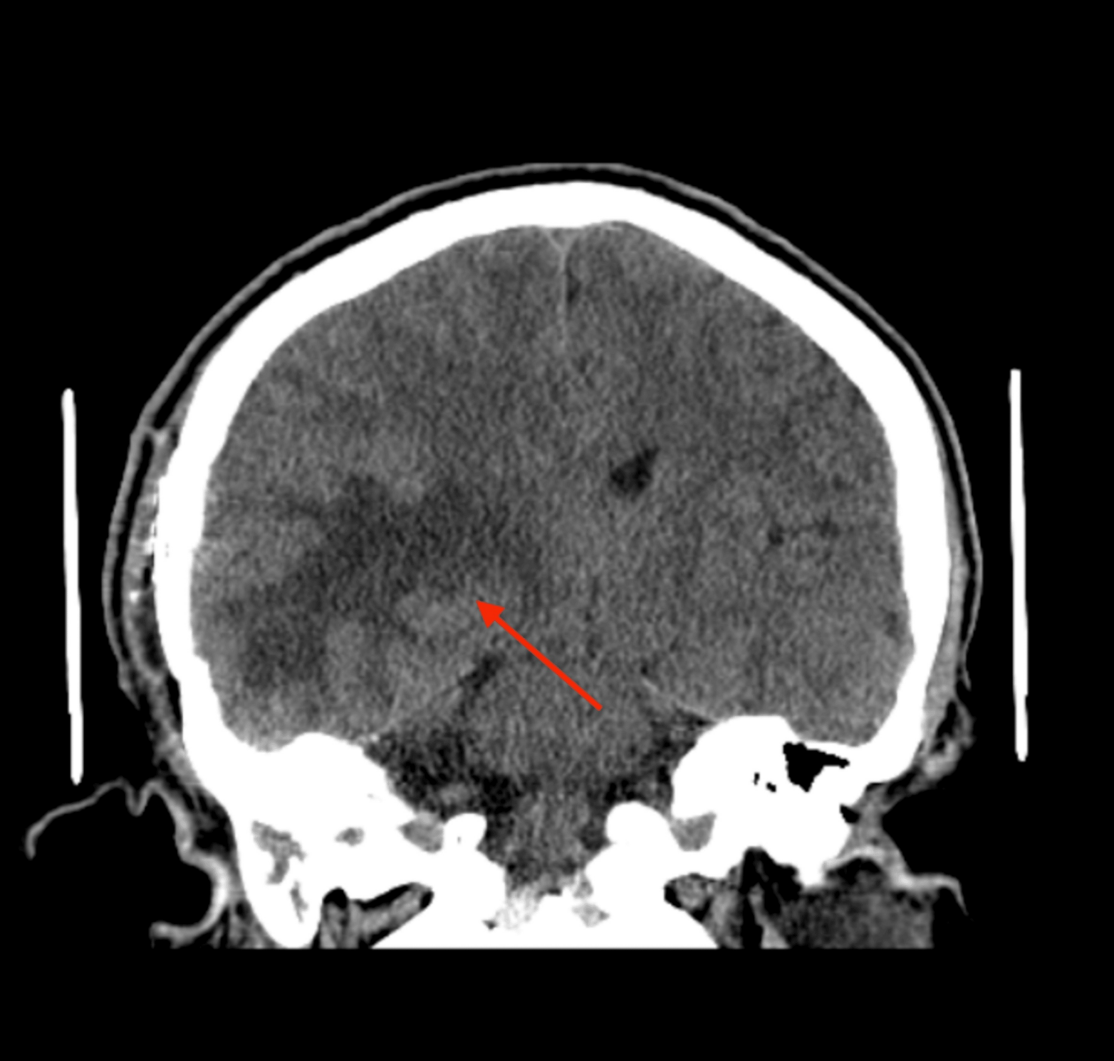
CT scan of the head (axial section) CT scan of the head (axial section) showing recurrent right temporal metastatic mass and 10 mm herniation to the left
